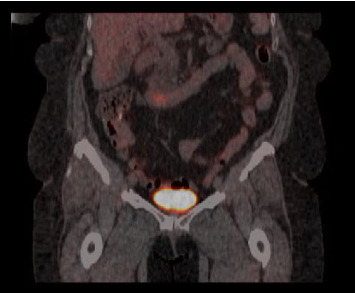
PET scan showing focal fluorodeoxyglucose (FDG) uptake in the third part of the duodenum.
